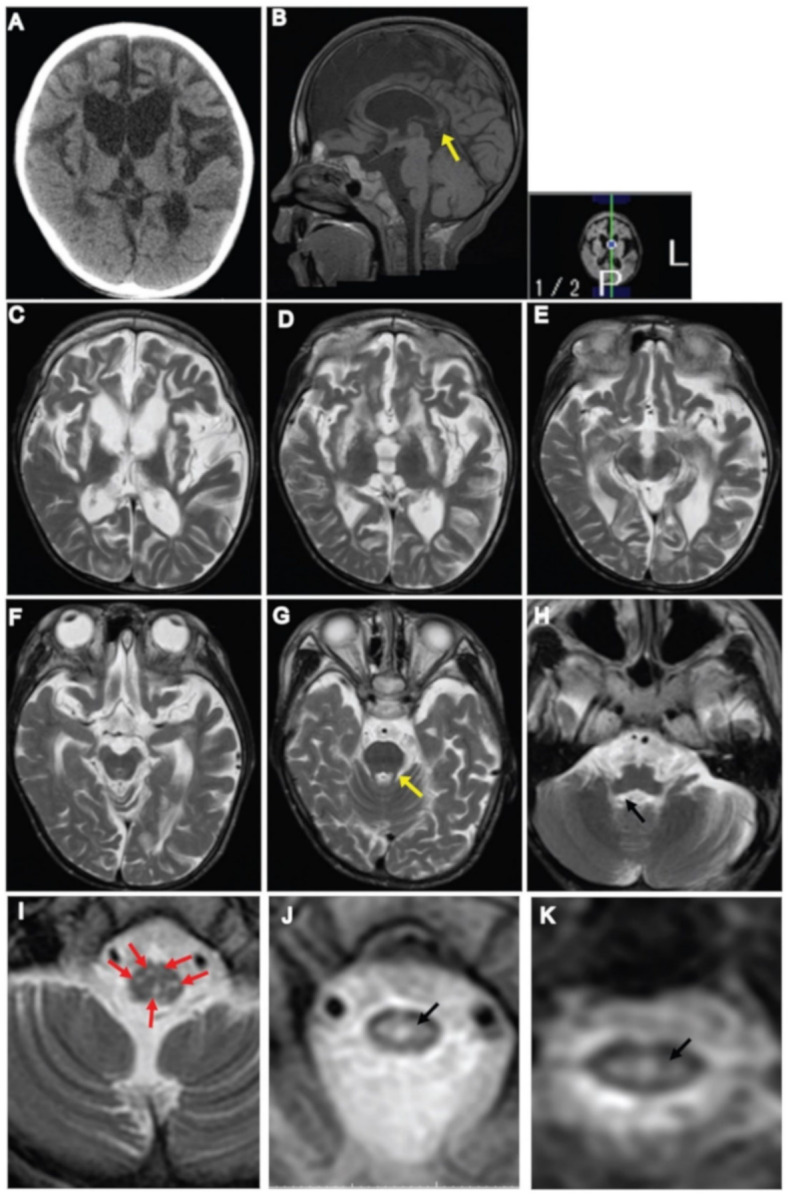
Neuroimaging findings at 13 years of age. (A) CT scan of the brain showing cerebral atrophy and ventricular enlargement. (B) Sagittal T1-weighted image of the brain showing ventricular enlargement, thinning of the corpus callosum with foci in the posterior body of the corpus callosum (arrow). (C–E) Axial T2-weighted images of the brain showing signal changes in the periventricular and frontal white matter, the putamen, caudate nucleus, corpus callosum, and anterior limb of the internal capsule. (F–I) Within the brainstem: (F) no signal abnormality was observed in the midbrain; (G) superior cerebellar peduncle (yellow arrow); (H) inferior cerebellar peduncle (black arrow); (I) T2-axial images of the medulla showing spotty high signal in the medulla (arrows), however pyramids and medial lemniscus decussation were preserved. (J,K) T2-axial image of upper cervical spinal cord showing high intensity area in the central part of the spinal cord (arrow).
